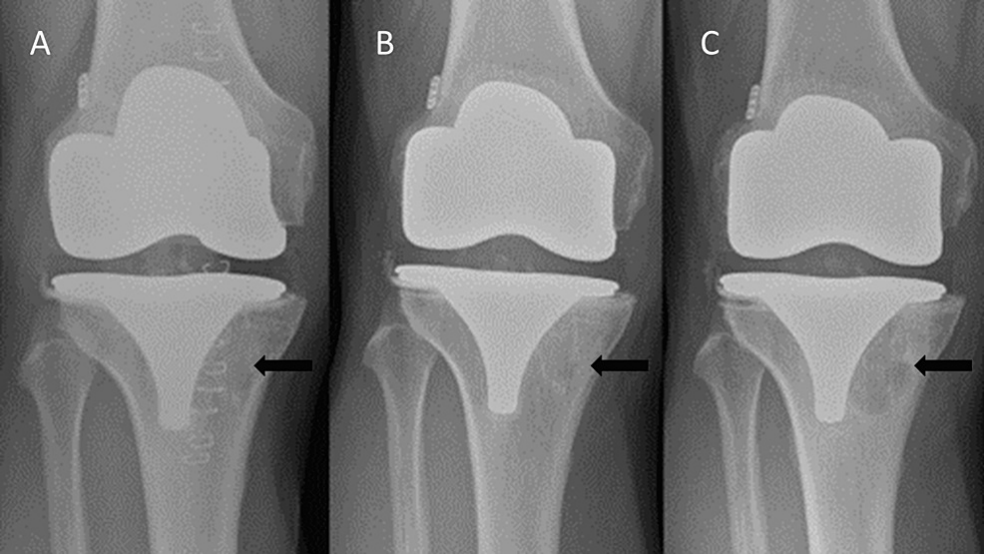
Series of radiographs depicting progressive osteolysis. Osteolysis in comparison depicted by an arrow on antero-posterior radiographs taken in (A) February 2016, (B) July 2017 and (C) June 2019.
